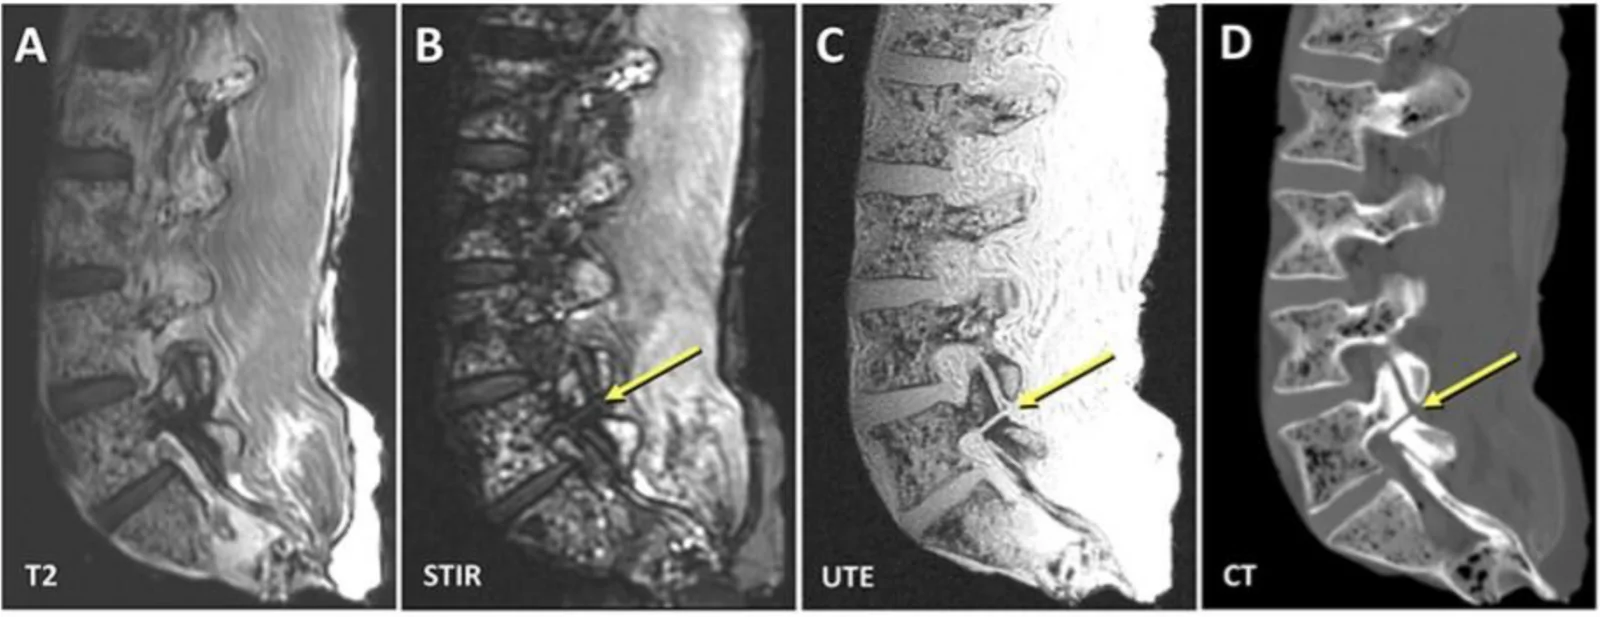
A 42-year-old female with pars fracture not detected on (A) conventional T2 imaging. The short tau inversion recovery (STIR) sequence (B) shows pars defect to some extent. Both the ultrashort time-to-echo (UTE) (C) and the CT (D) images clearly depict fracture within ~ 1 mm width (fracture indicated by yellow arrows) [42]; reproduced from Finkenstaedt et al. [42], with permission
